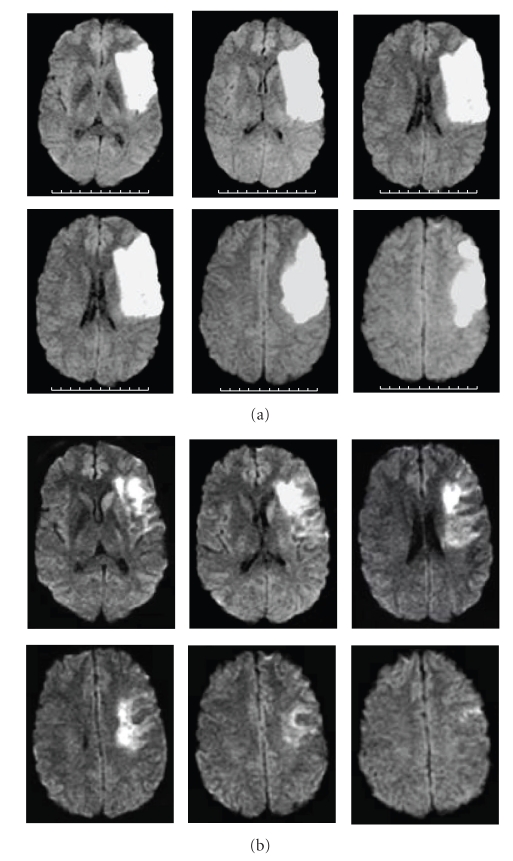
MRI diffusion-weighted images showing left superficial MCA infarct. (a) 24 hours after stroke onset, (b) 5 days after stroke onset.
